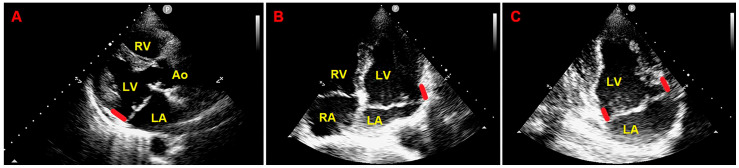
Transthoracic echocardiography. MAD assessment at end-systole from the parasternal long-axis view (A), from the apical four-chamber view (B), and from the apical two-chamber view (C). The bold red line indicates the MAD distance. Ao, aorta; LA; left atrium; LV, left ventricle; RA, right atrium; RV, right ventricle.
